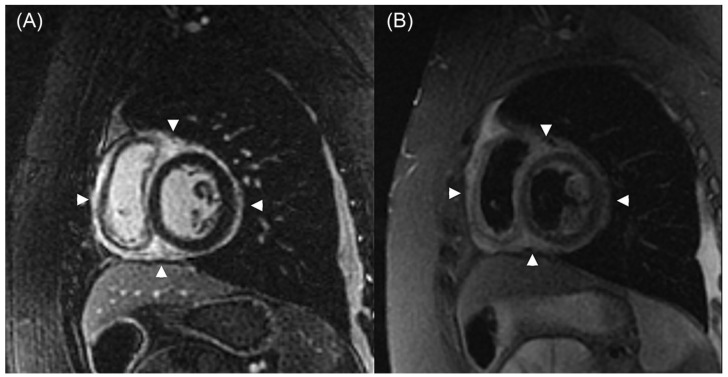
CMR shows a widespread epicardial distribution of LGE in the setting of graft dysfunction. In Panel (A), a short-axis, post-contrast inversion recovery gradient echo image at the midventricular level reveals diffuse epicardial enhancement in both ventricles (arrowheads). Panel (B) shows a corresponding short-axis T2-weighted triple inversion recovery fast spin-echo image, displaying increased signal intensity (arrowheads) in the same region, consistent with myocardial edema. Adapted from Anand et al., 2024 [47]. CMR—cardiac magnetic resonance imaging; LGE—late gadolinium enhancement.
